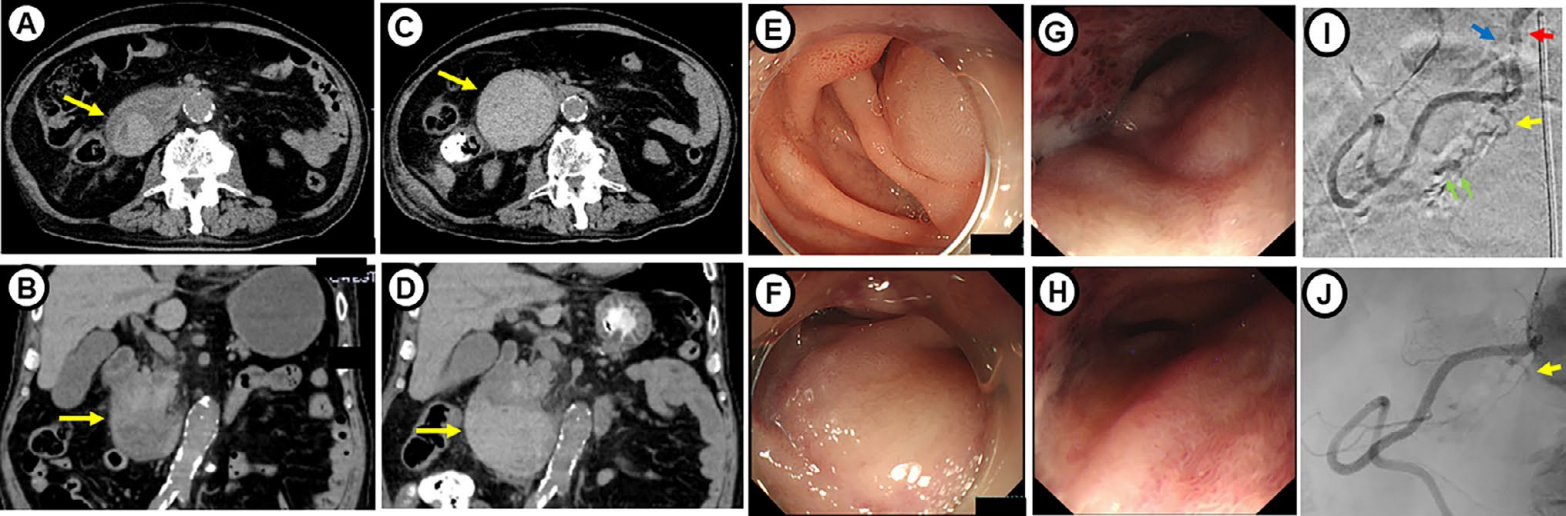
(A, B) CT findings revealed a mixed hyperdense and hypodense mass in the second portion of the duodenum (arrow). (C, D) CT findings obtained after 3 days revealed that the hematoma had become enlarged (arrow). (E, F) Esophagogastroduodenoscopy showed an intramural duodenal hematoma obstructing the second portion of the duodenum (standard endoscopic view obtained with GIF‐Q260J, diameter 9.9 mm, Olympus Corp., Tokyo, Japan). (G, H) Findings obtained deeper into the duodenum (thin endoscopic view obtained with GIF‐XP290N, diameter 5.8 mm, Olympus Corp, Tokyo, Japan). (I) Angiography findings. Red arrow, gastroduodenal artery; yellow arrow, anterior superior pancreaticoduodenal artery (ASPDA); blue arrow, posterior superior pancreaticoduodenal artery; extravasation from ASPDA branches was noted (green arrows). (J) Findings obtained following embolization of the ASPDA showed no evidence of active bleeding.
